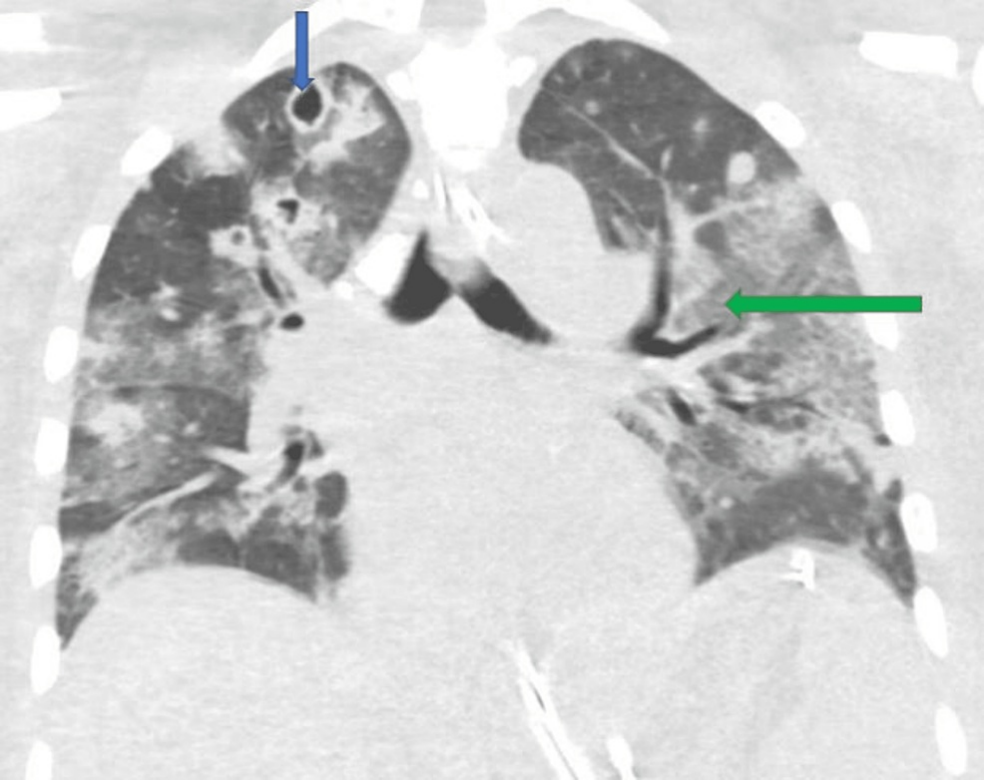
Computed tomography (CT) scan of the chest (day 1) showing multifocal confluent consolidations and cavitary lesions with superimposed diffuse ground-glass opacities. The blue arrow demonstrates a right apical cavitary lesion while the green arrow shows a broad area of ground-glass opacity.
